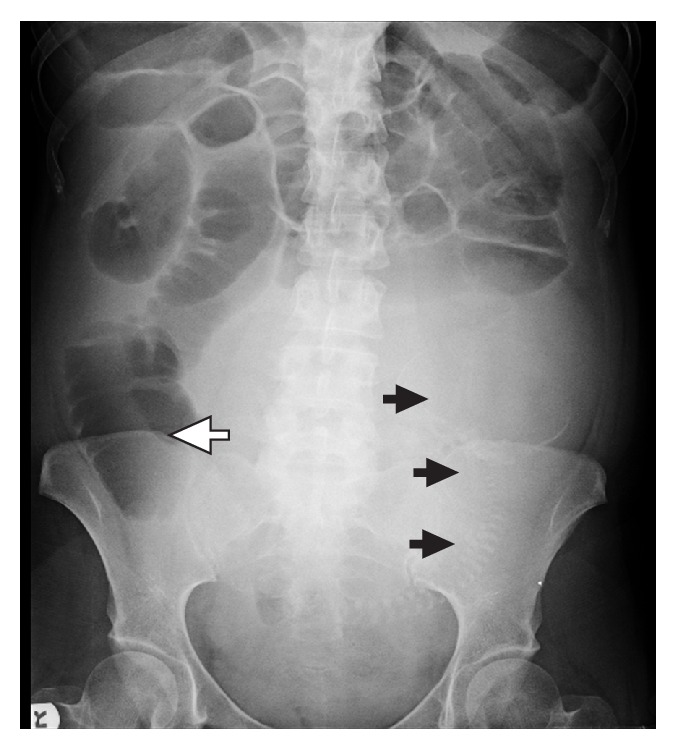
Preoperative abdominal X-ray film. Dilated loops of small abdomen in the upper abdomen. Dilated cecum or massively dilated small bowel loop in right lower abdomen (hollow arrow). Fetal bony silhouette is outlined with solid arrows.
